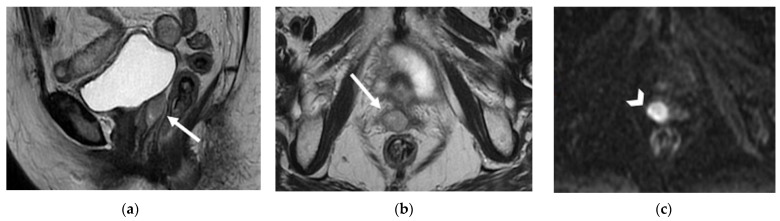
A 57-year-old patient who underwent radical hysterectomy with bilateral salpingo–oophorectomy for cervical cancer. (a) Sagittal and (b) axial FSE T2-weighted images show a nodular hyperintense lesion in the vaginal cuff (arrows), consistence with relapse. (c) Diffusion-weighted image demonstrates highly restricted diffusion of the lesion (arrowhead) in relation to hypercellularity.
